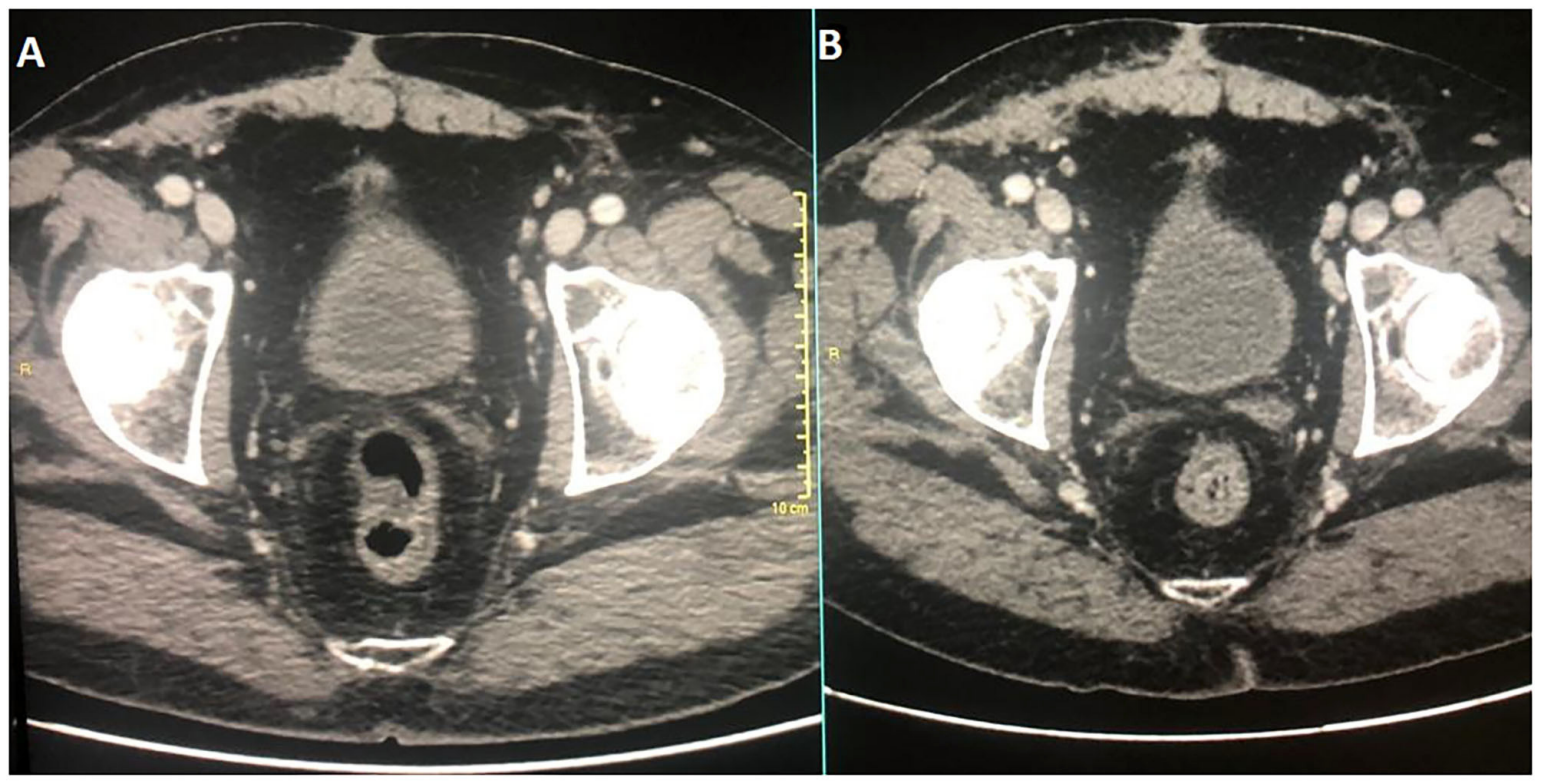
Diagnostic CT corresponding images at diagnosis and at therapy completion in (A,B), respectively.
